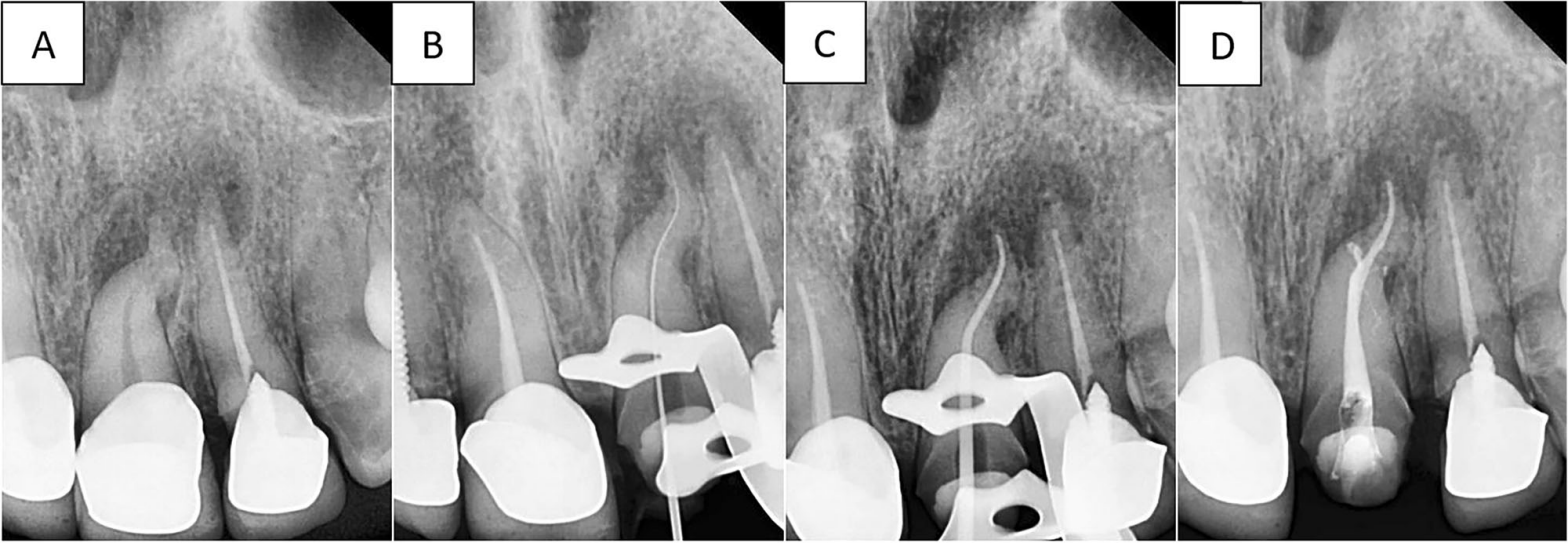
Endodontic procedure of maxillary necrotic central incisor in G1. Steps of the provided endodontic treatment in patients of G1 (A) diagnostic periapical radiograph, then (B) Working length determination, then (C) Cone Fit, and Finally (D) Final obturation.
